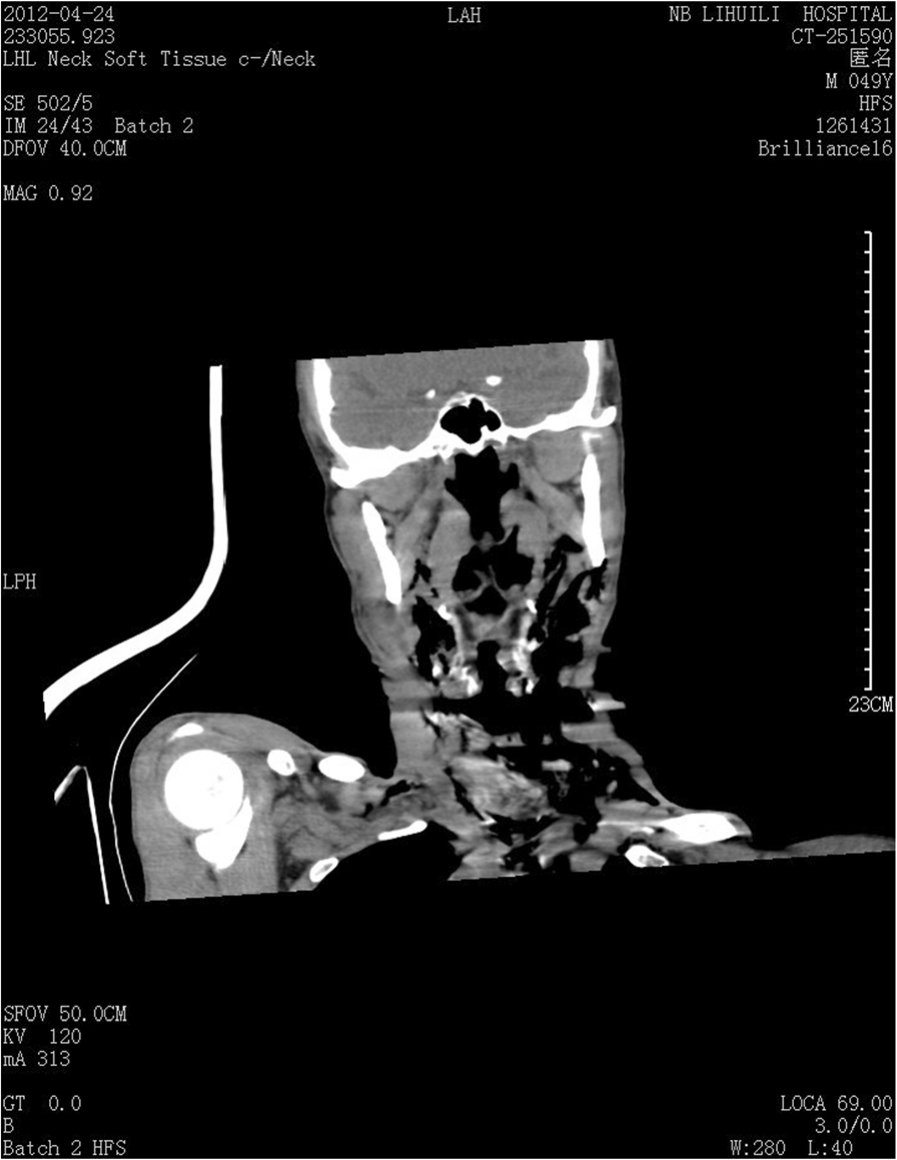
CT scan of the neck, showing disruption of the cervical trachea and widespread subcutaneous emphysema.
